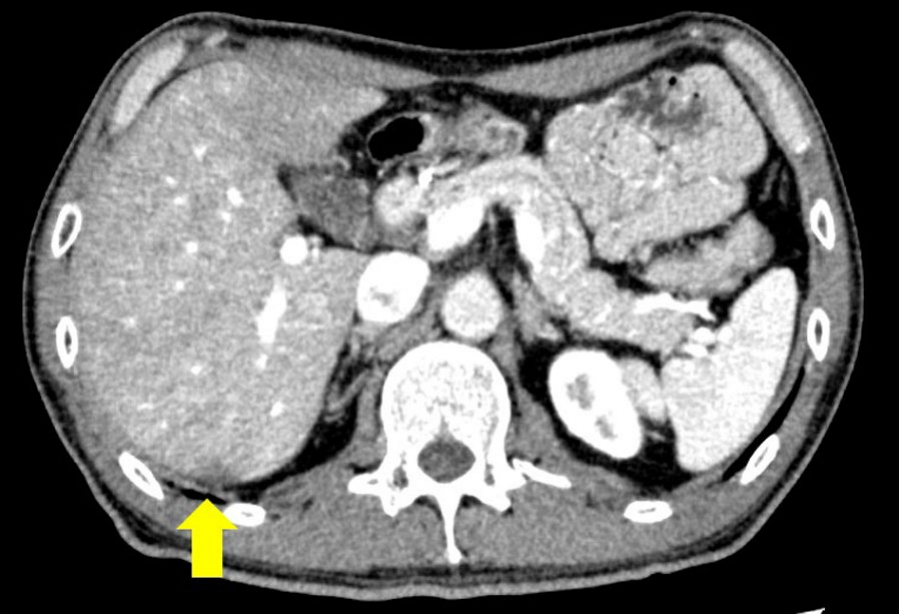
Result of CT after 4 courses of nivolumab combination therapy. CT demonstrating a reduction in the size of the liver metastasis to approximately 1 cm
